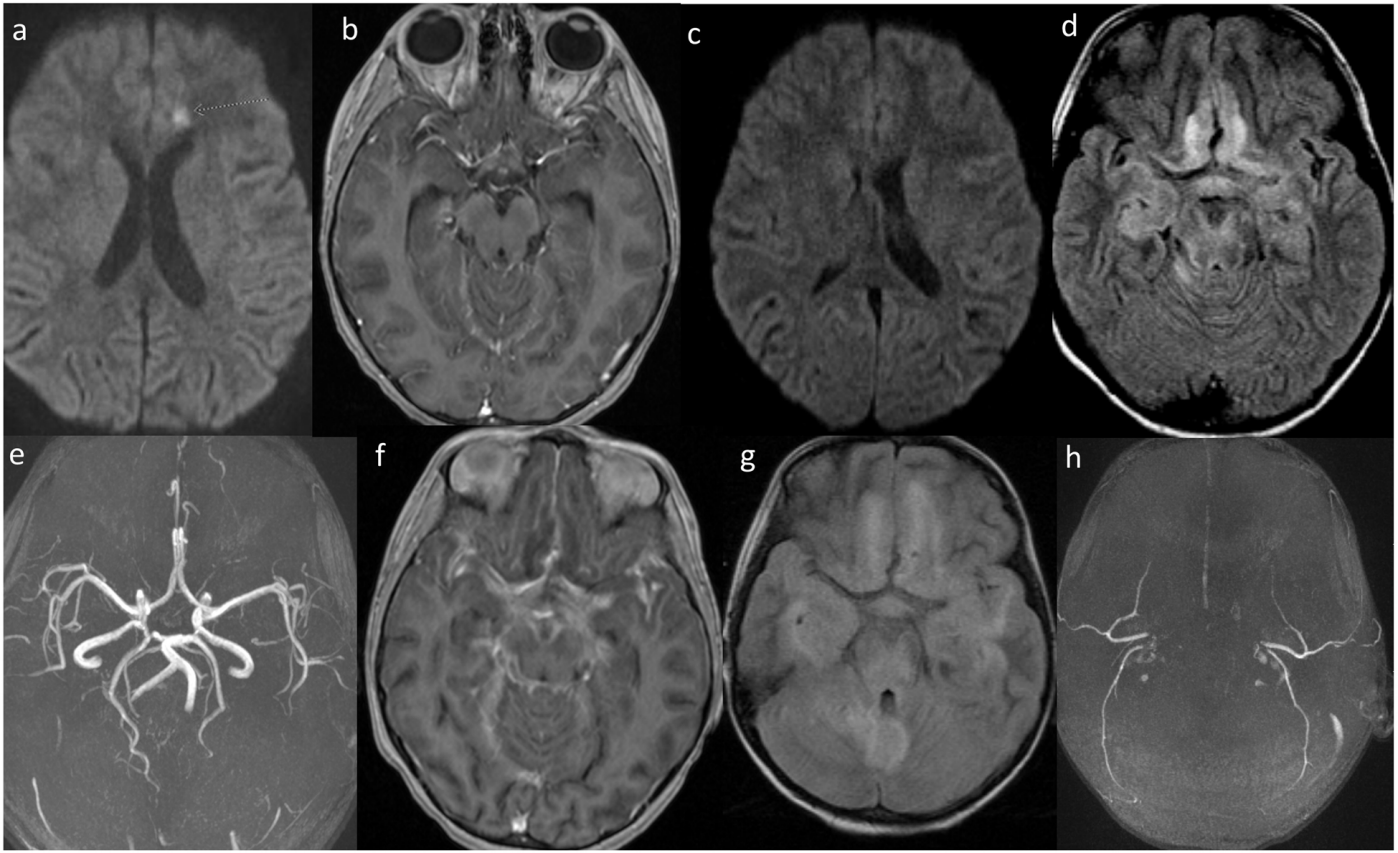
Selected sequential MRI/MRA images. a Diffusion-weighted image shows a 7 mm area of restriction diffusion in the left anterior frontal lobe subcortical white matter (arrow), day 12 of illness; b T1-weighted image with gadolinium showing mild leptomeningeal enhancement along the tentorium, day 12; c Diffusion-weighted image showing no restriction diffusion in the left anterior frontal lobe subcortical white matter, day 16; d T2 FLAIR shows abnormal increased signal mainly involving the midbrain, bilateral deep frontal, and bilateral medial temporal areas, day 16; e 3-D time of flight MRA shows a normal circle of Willis, day 16; f T1-weighted image with gadolinium showing diffuse leptomeningeal enhancement, day 16; g T2 FLAIR showing extensive grey matter lesions involving the deep frontal lobe, temporal lobe, midbrain, and cerebellar vermis, with no obvious cerebellar involvement, day 30; h 3-D time of flight MRA showing no flow in intracranial vessels with flow in extracranial vessels, day 30
